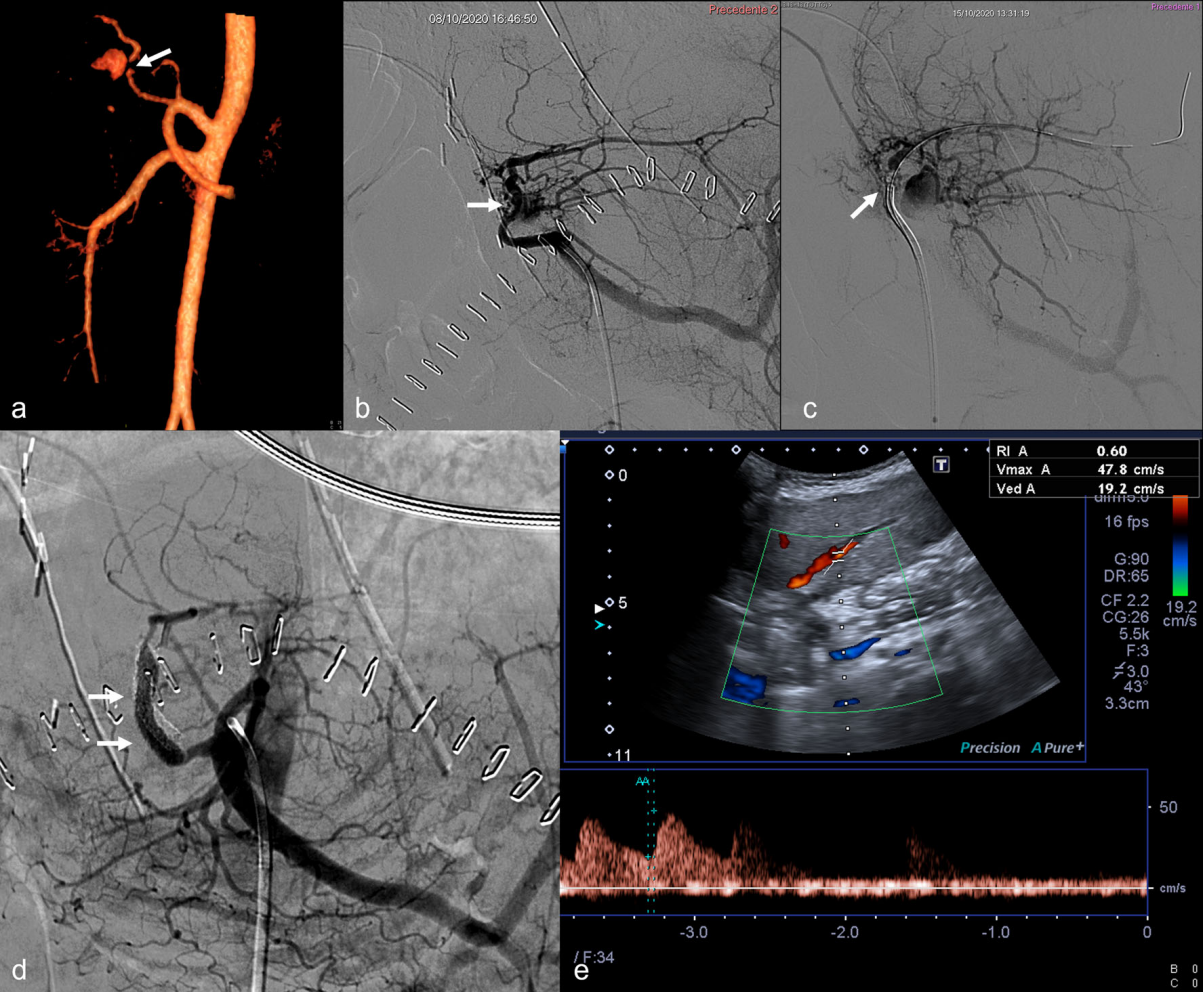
Acute hepatic artery stenosis and pseudoaneurysm in a 3-year-old female (case #7) 2 days after meso-rex bypass creation due to extrahepatic portal vein thrombosis after split liver transplantation. a CT-angiography 3D rendering depicts the anatomy of the hepatic artery with stenosis (arrow) and pseudoaneurysm located within the liver. b Selective angiogram of the hepatic artery shows the pseudoaneurysm (arrow) involving the origin of the arterial branch for segment 3; c selective angiogram performed after placement of the guiding catheter (Envoy 5 F) tip (arrow) across the stenosis and the pseudoaneurysm’s neck; d final angiogram performed from the celiac trunk after coaxial stent grafts (arrows, PK Papyrus 3 × 20 mm + 4 × 20 mm) placement shows regular opacification of the hepatic artery and its intrahepatic branches with pseudoaneurysm not opacified. d Follow-up color-Doppler ultrasound shows patency of an intrahepatic branch of the hepatic artery with a normal waveform
